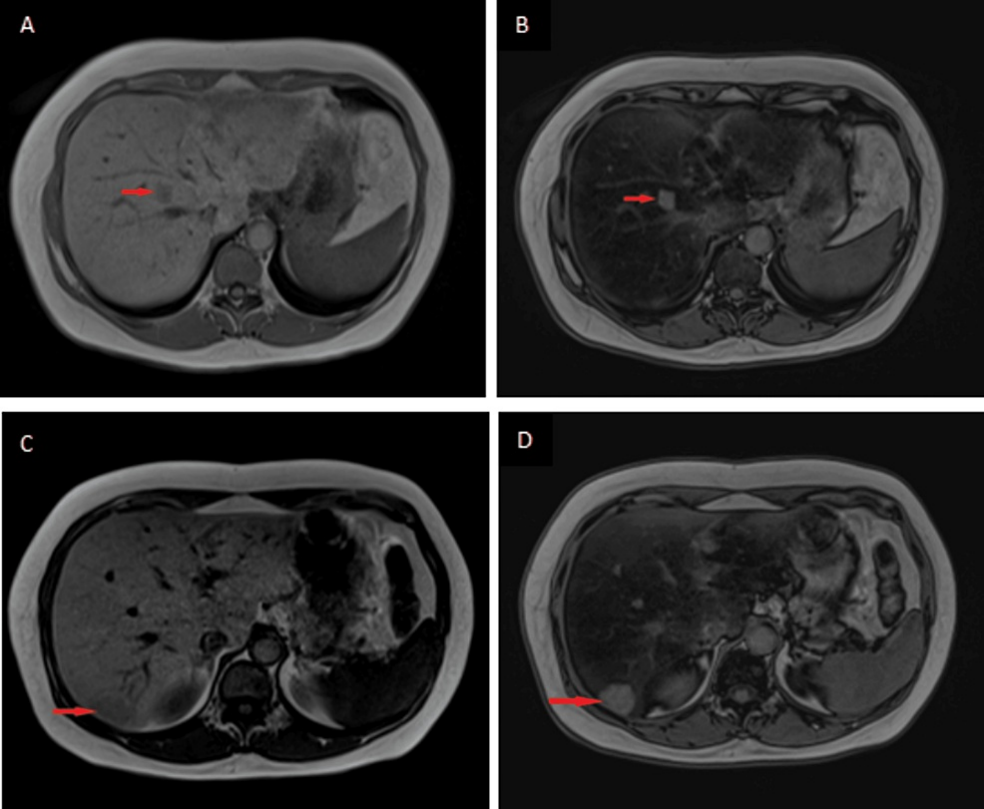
Axial T1W In-phase gradient-echo MR images (A and C) and axial Opposed-phase gradient-echo MR images (B and D) at two levels within the upper abdomen reveal a signal drop of the liver with focal areas (red arrows) of a maintained signal appearing relatively hyperintense to the fatty liver in the opposed-phase images.
